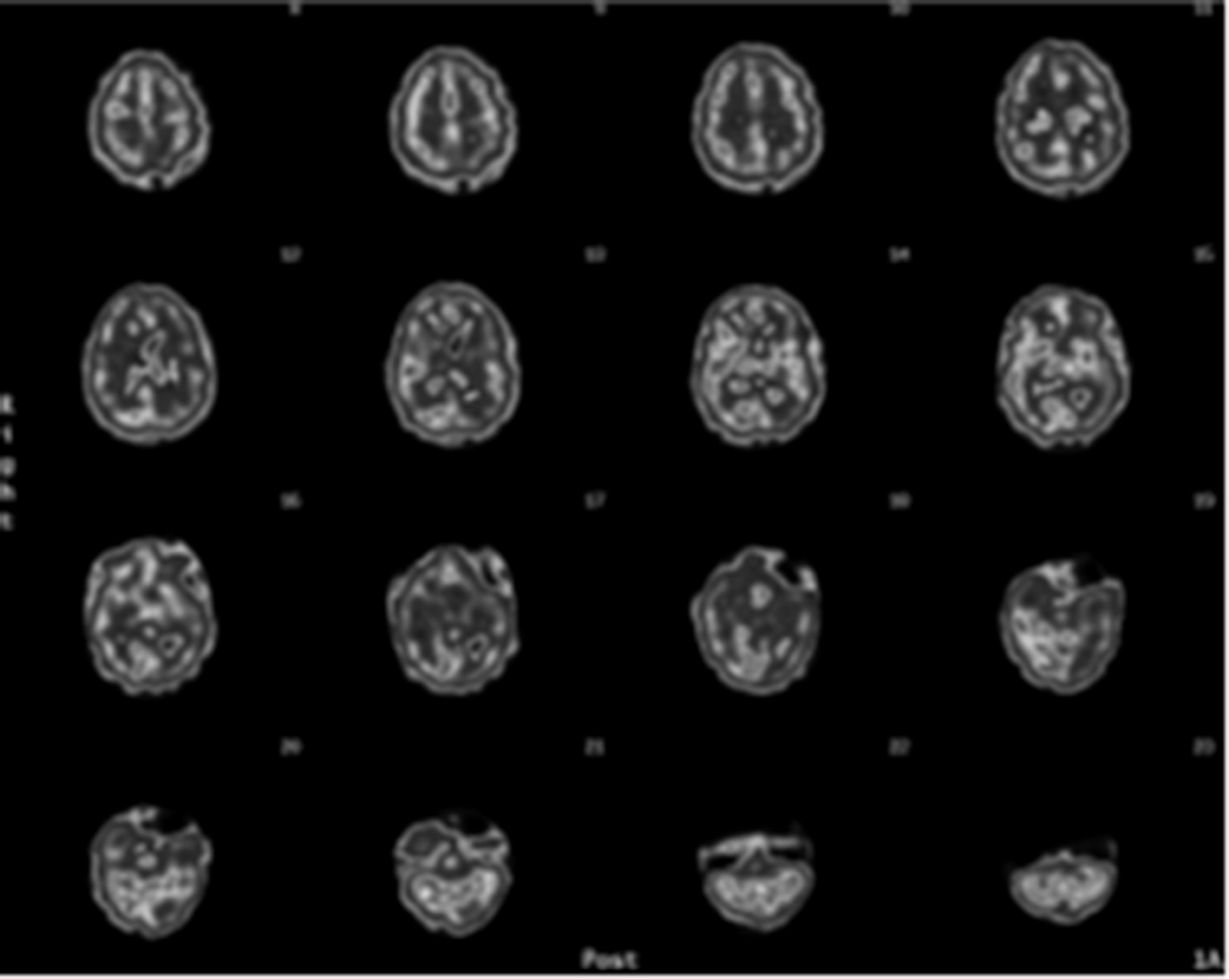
Typical gray scale SPECT rendered transaxial sliced images.
